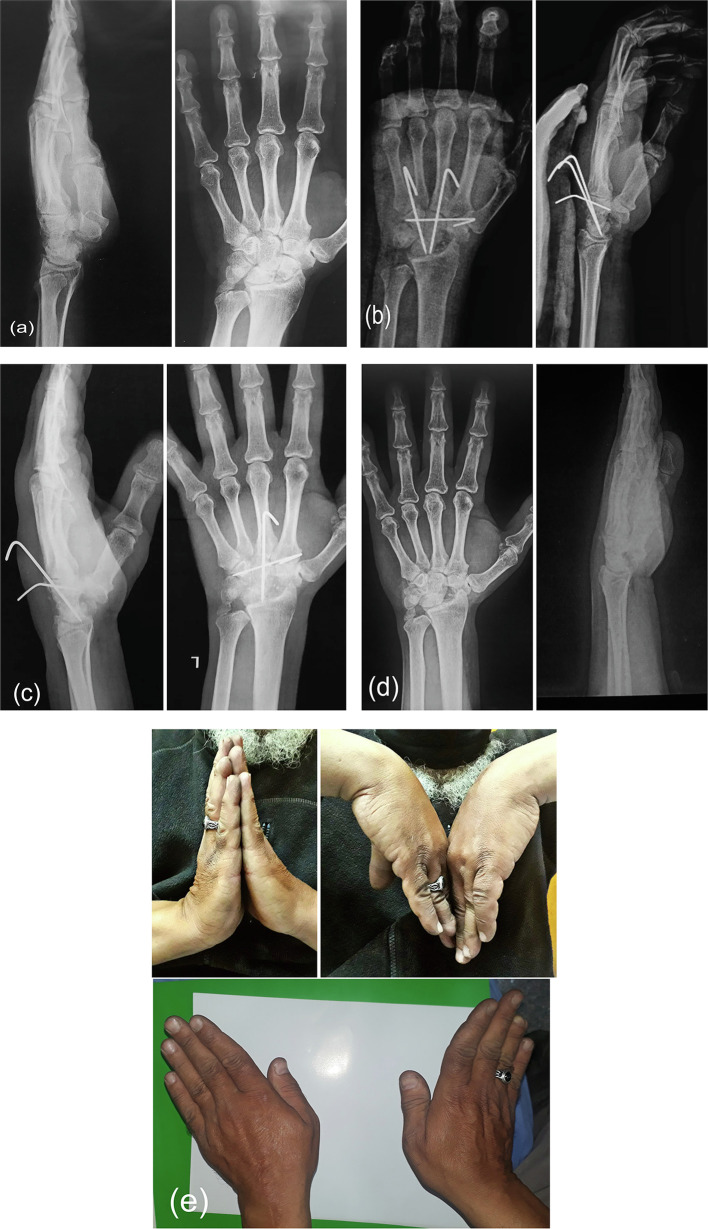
Case from 3CFT. (a) Preoperative X rays, show SNAC stage III; (b) Immediate postoperative X-rays, with correction of DISI deformity on lateral radiographs; (c) At 8 weeks follow-up, with good union between carpal bones; (d) On of the wires was removed as it became loose; (e) After one-half year follow-up.
